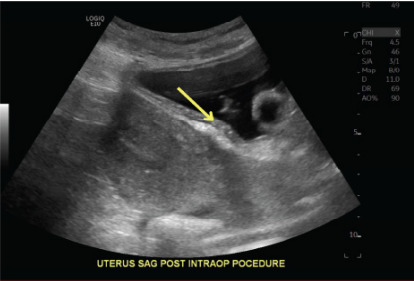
Transvaginal ultrasound showing uterus after products of conception were removed (arrow).
